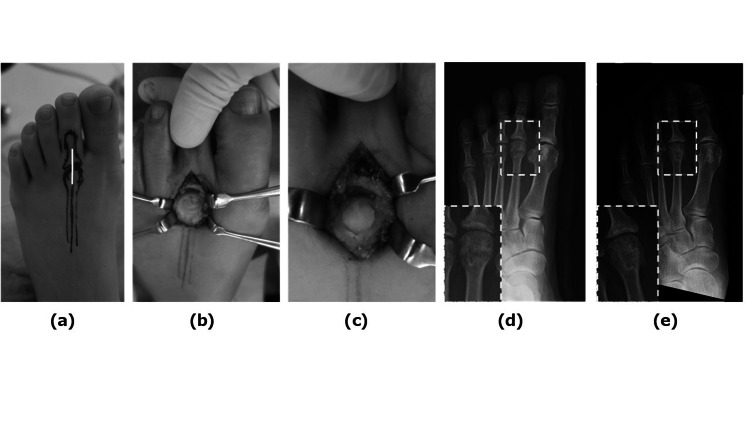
Case 1—Intraoperative photographs (a-c) and postoperative radiographs (d-e) (a) A longitudinal skin incision was made directly over the MTPJ (the incision line is indicated by a white line) (b) Severe deficiency observed in the cartilaginous surface (c) Results following OAT (d) AP view of the foot immediately postoperatively (the area enclosed by a dotted square is enlarged and shown in the lower left) (e) Radiograph one year postoperatively: Healthy osteochondral column with unchanged joint space size (the area enclosed by a dotted square is enlarged and shown in the lower left) AP: Anteroposterior; MTPJ: Metatarsophalangeal joint; OAT: Osteochondral autograft transplantation
